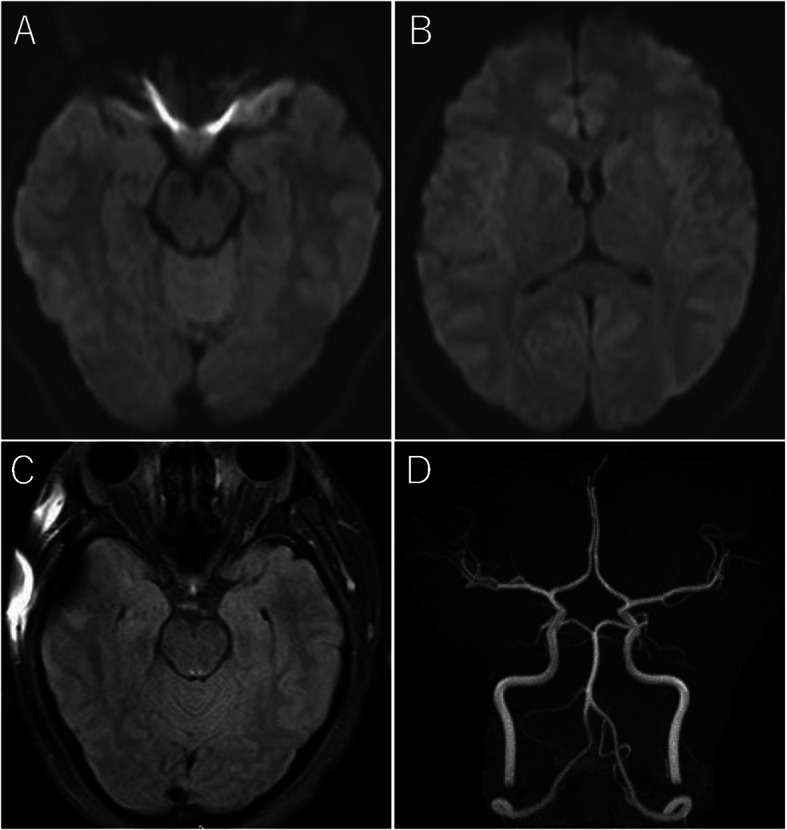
a, b Case 1: Diffusion-weighted magnetic resonance images: The brain shows no abnormalities. c, d Case 1: Fluid-attenuated inversion-recovery (FLAIR) images and magnetic resonance angiography (MRA) show no abnormalities
